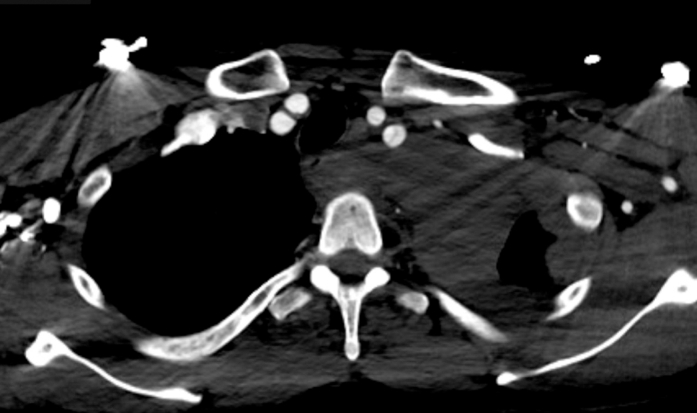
Axial Computed Tomography Demonstrating Acute Dissection of the Left Subclavian and Left Common Carotid Arteries
